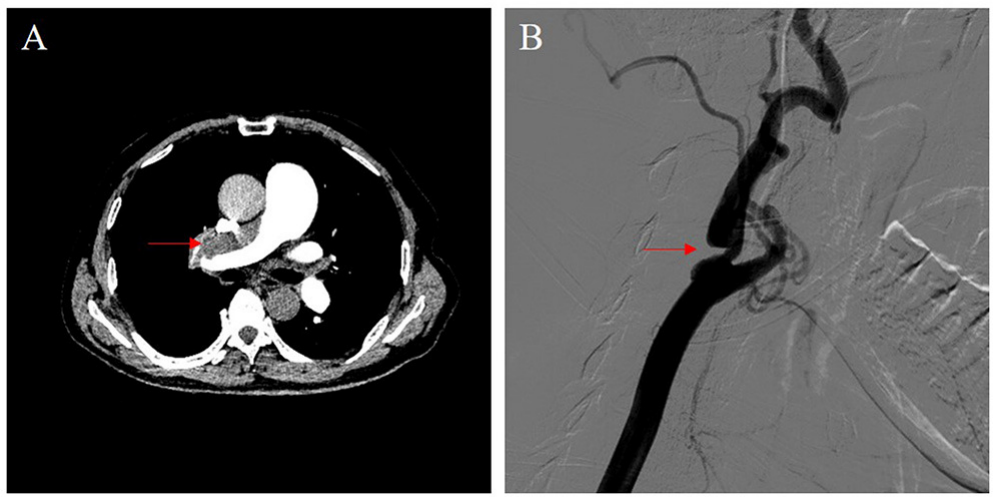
Imaging data. (A) Pulmonary computed tomography angiography demonstrated a filling defect located in the main trunk of the right pulmonary artery (red arrow). (B) Carotid artery angiography showed severe stenosis of the right internal carotid artery (red arrow).
